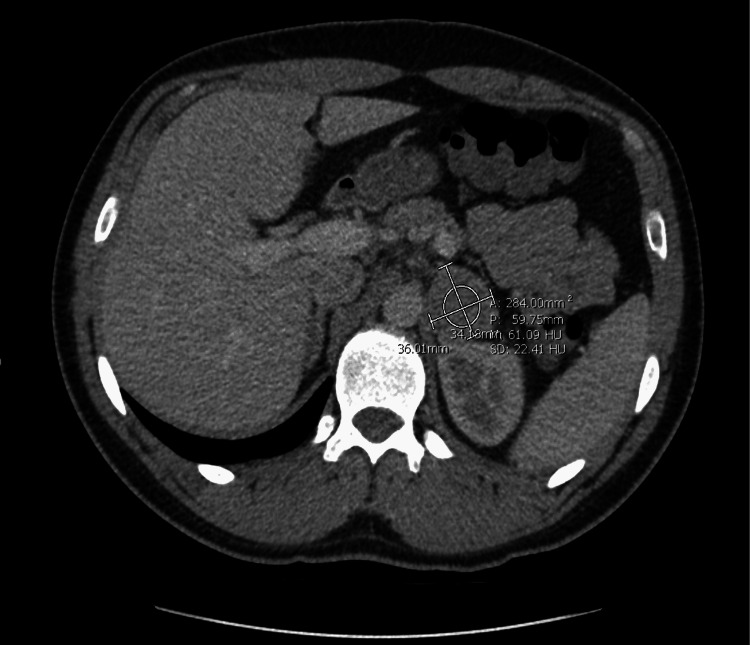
CT adrenal with and without contrast showing a 3.6-cm left-sided adrenal mass.
